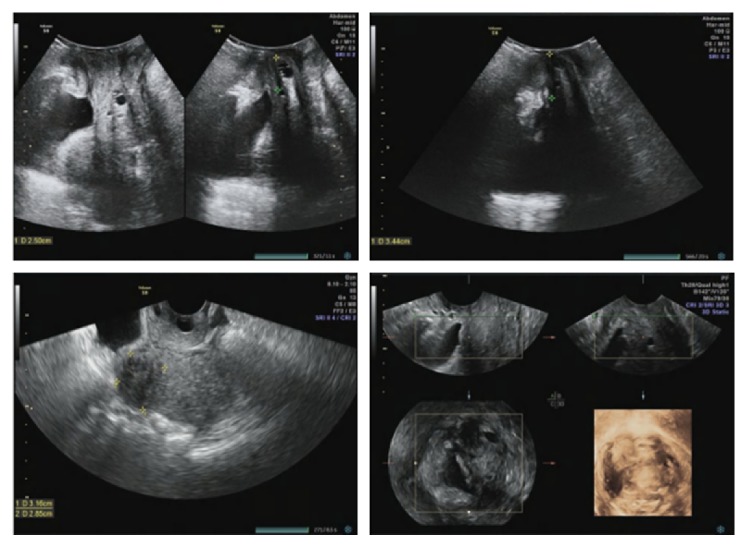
Pelvic floor four-dimensional ultrasound of Case 2. Pelvic floor four-dimensional ultrasound indicated that residual urine was 0 ml, thickness of detrusor was normal, internal orifice of urethra was closed, posterior angle of bladder was intact, and there was no dark area of liquid and scattered point of calcification around urethra in quiescent condition. CDFI revealed that sparse color flow signals were seen around the urethra, the bladder neck was 19 mm above the pubic symphysis, the uterus was 17 mm above the pubic symphysis, and ampulla portion of rectum was located at the pubic symphysis. Bladder neck displacement was 15 mm, bladder neck was located 9 mm below the pubic symphysis, posterior angle of bladder was intact, the uterus was 35 mm below the pubic symphysis, ampulla portion of rectum was located at the pubic symphysis, rectocele was not seen, and anal sphincter was complete in Valsalva.
